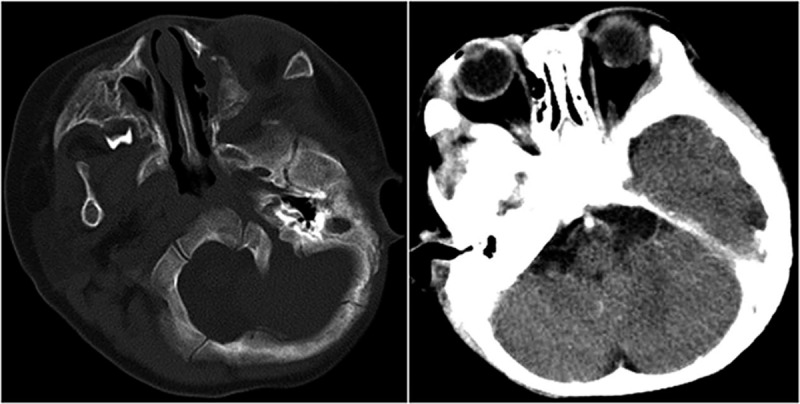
CT scans revealed that the tissue mass in nasal cavity was invisible. CT = computed tomograpghy.
